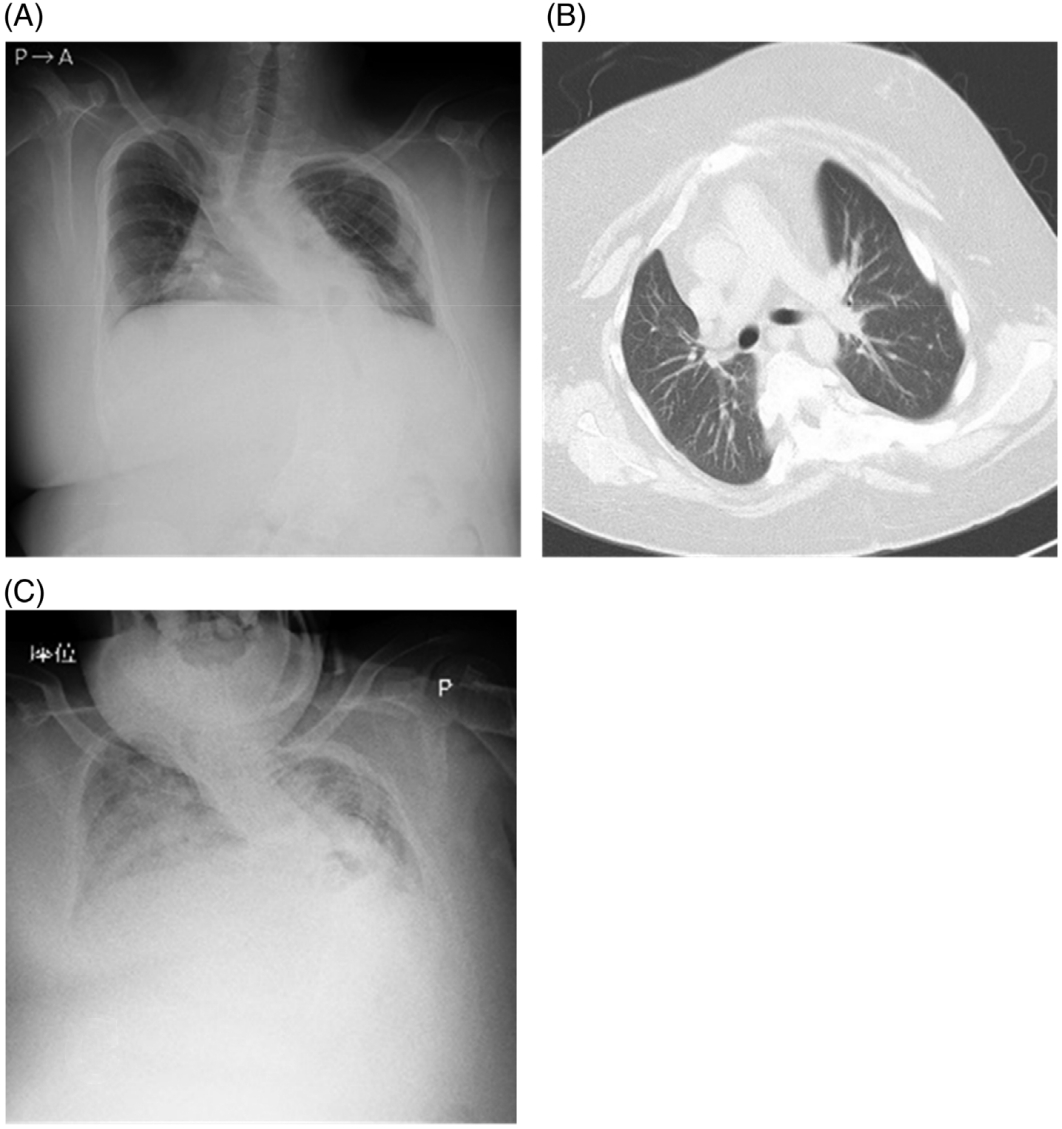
Chest X‐ray and computed tomography (CT) of case 1 (A) Chest X‐ray on admission showed the curvature of the spine with thoracic asymmetry due to scoliosis. (B) CT on admission revealed thick subcutaneous fat surrounding the thorax and no infiltration in the pulmonary fields. (C) Chest X‐ray on day 11 showed diffuse infiltration of the bilateral lungs due to pneumonia and congestive heart failure
